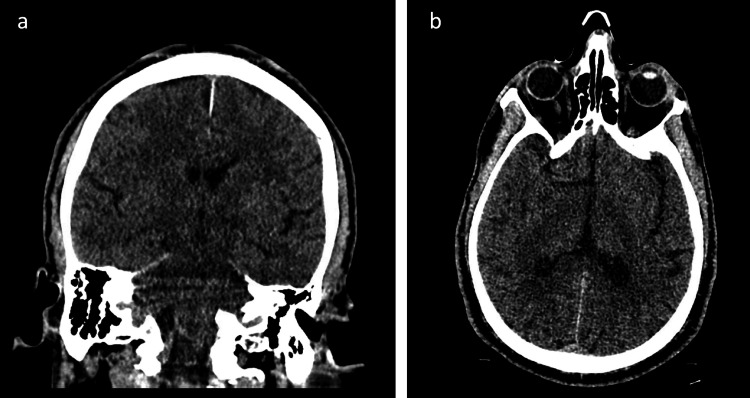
Coronal (a) and axial (b) non-contrast computed tomography (CT) images taken at arrival to the emergency department
